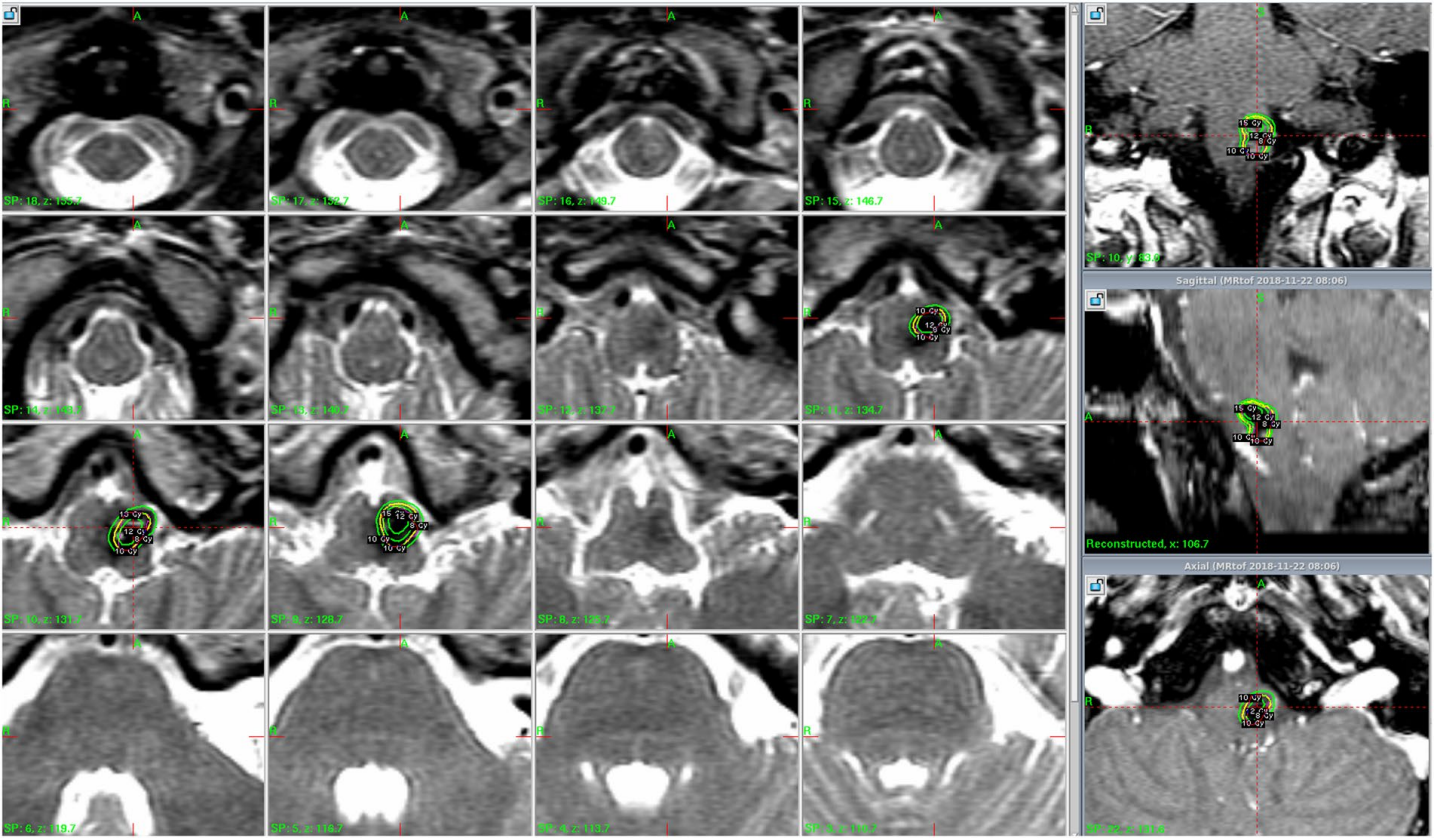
A 27-year-old female presented with a sudden onset of left limb weakness and numbness, gait disturbance, diplopia, and facial numbness for one week. A CCM with associated hemorrhage that measured 3.5 mL in volume was found in the medulla. T2-weighted imaging on MRI demonstrated a hemosiderin ring around the CCM. The CCM was treated using GKS with a margin dose of 10 Gy at a 55% isodose level. The CCM was controlled for up to 63 months.
